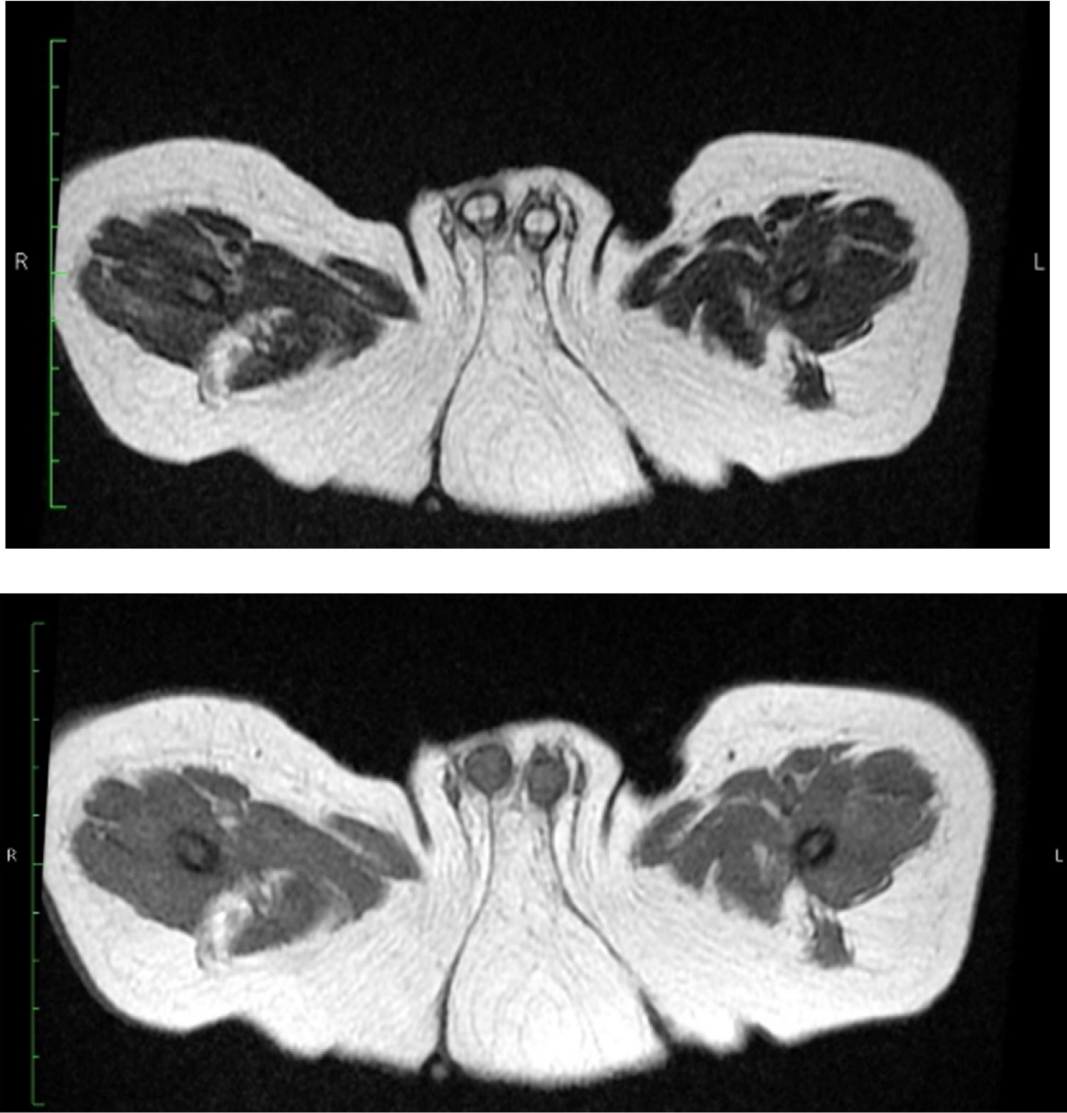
Axial T1-weighted and T2-weighted MR image show the penises and urethrae structures clearly; the presacral mass is seen at the posterior side between the anal dimples.
